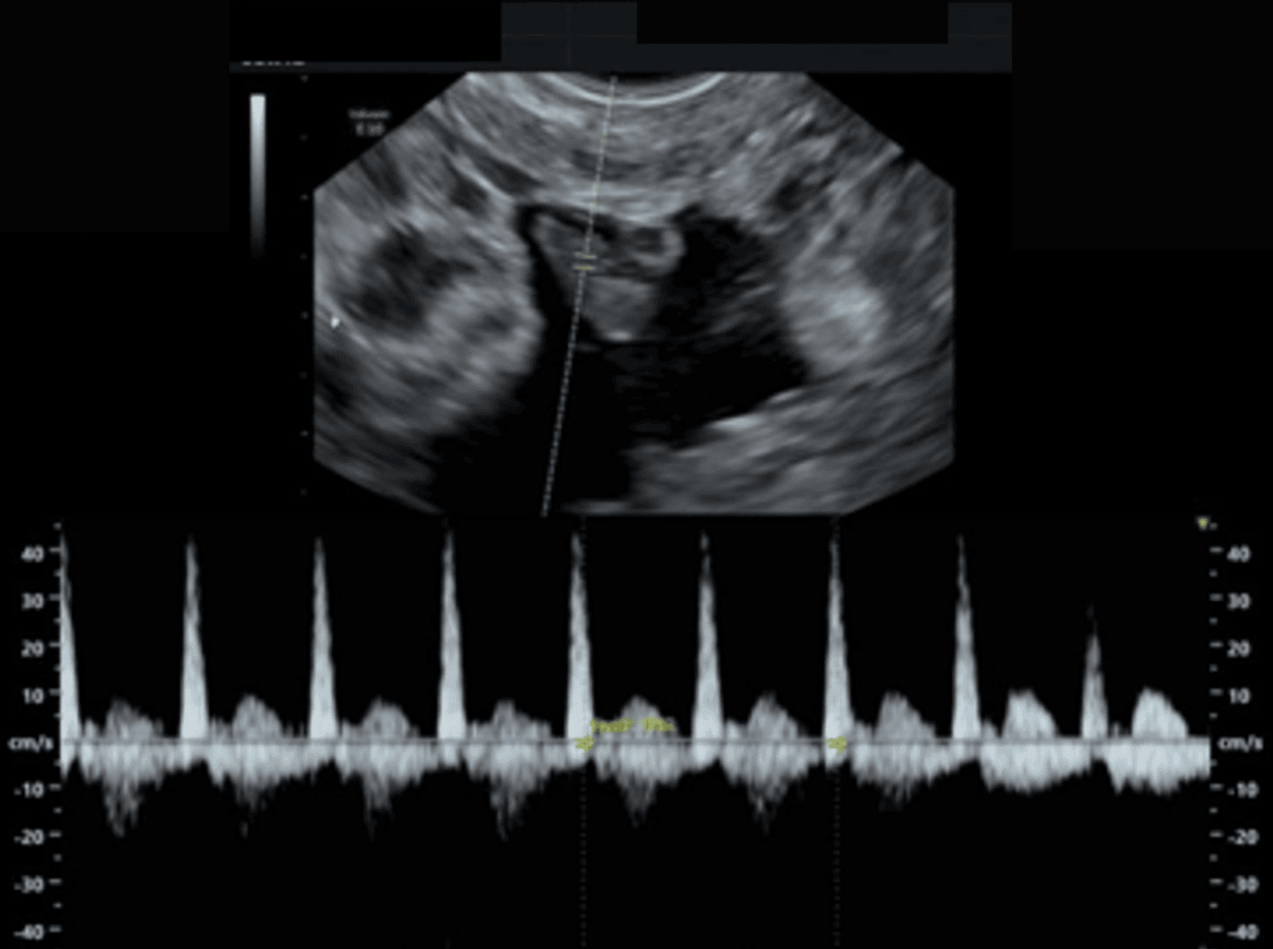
Transvaginal ultrasound fetal echo
